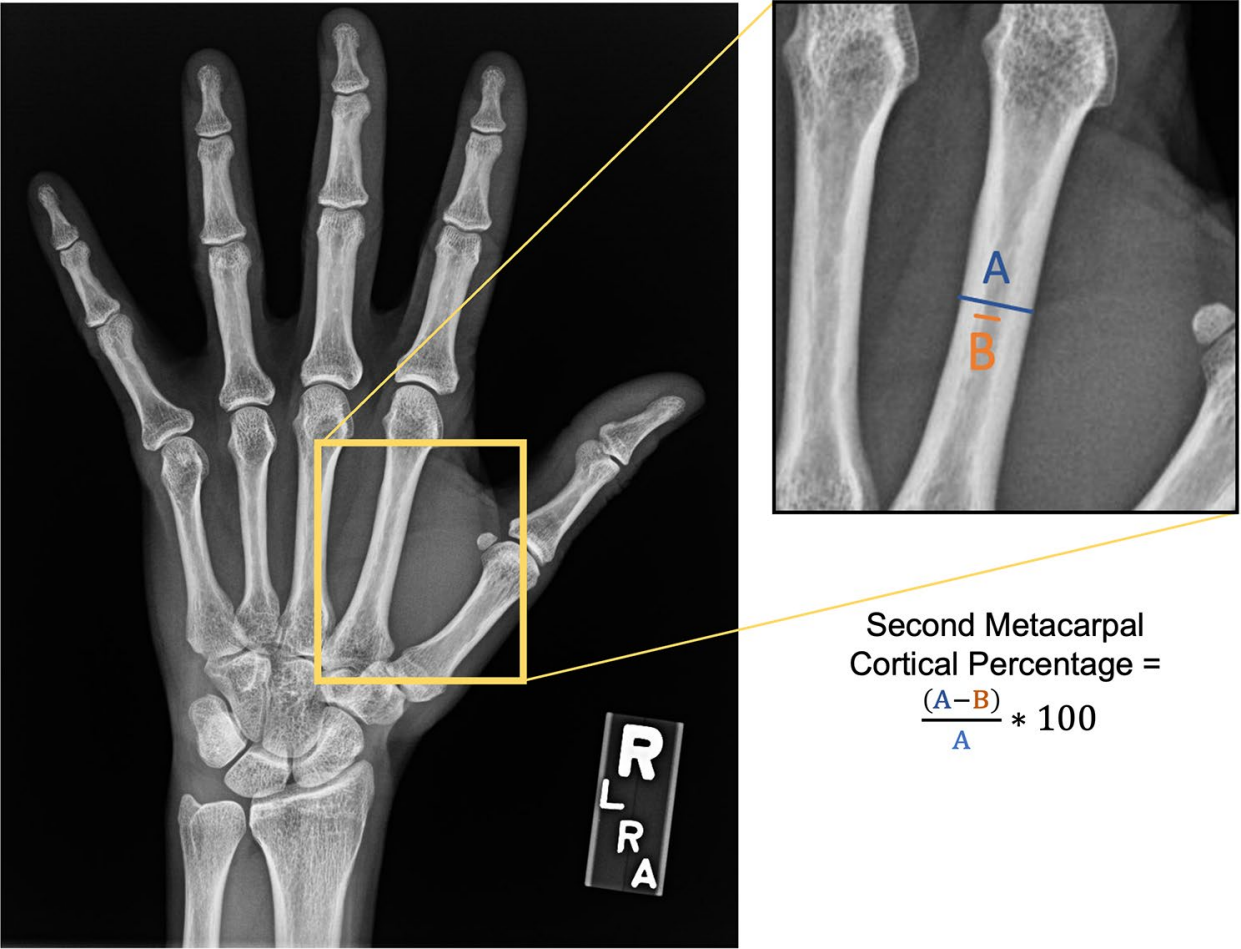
Measurement of second metacarpal percentage using hand radiographs. (A) measures the cortical diameter at the isthmus and (B) measures the intramedullary diameter
